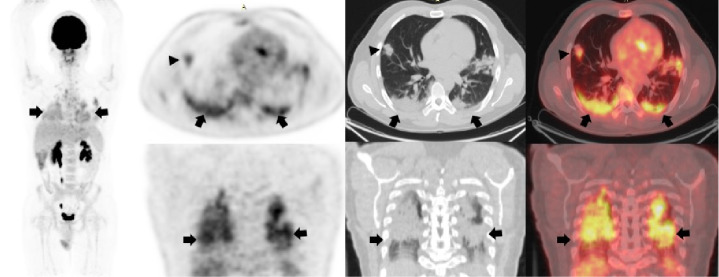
PET/CT F18-FDG que evidenció en el tórax (flechas negras) múltiples opacidades nodulares y pseudonodulares en ambos parénquimas pulmonares, así como opacidades alveolares con broncograma aéreo, multilobares en ambas bases, algunas dispersas en los lóbulos superiores y la língula, con SUVmáx de 3,8 dominante en la base derecha, y opacidades nodulares mal definidas, con broncograma, dispersas aleatoriamente, y nódulo irregular hipermetabólico en el segmento lateral del lóbulo medio (cabeza de flecha) de 24 mm de diámetro y densidad de partes blandas, con SUVmáx de 3,3.
